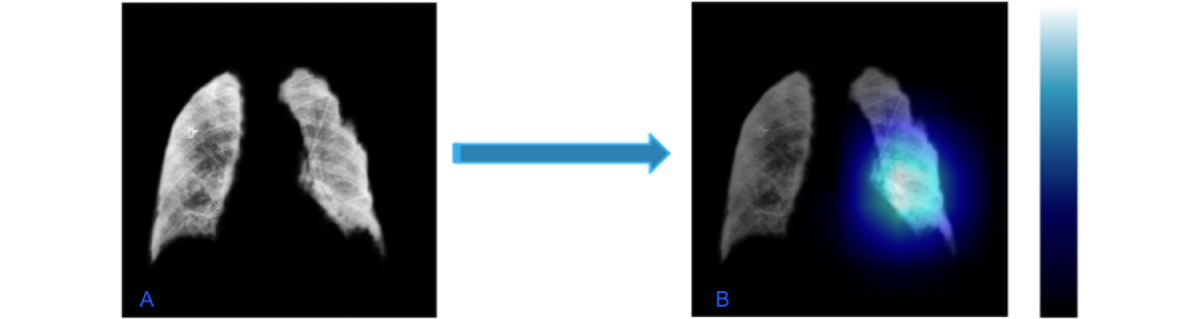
(A) Example of a segmented lung from the last chest radiograph of an intubated patient. (B) The most important features (pixels) predicting intubation are highlighted in the class activation map calculated by gradient-weighted class activation mapping projected on the image.
